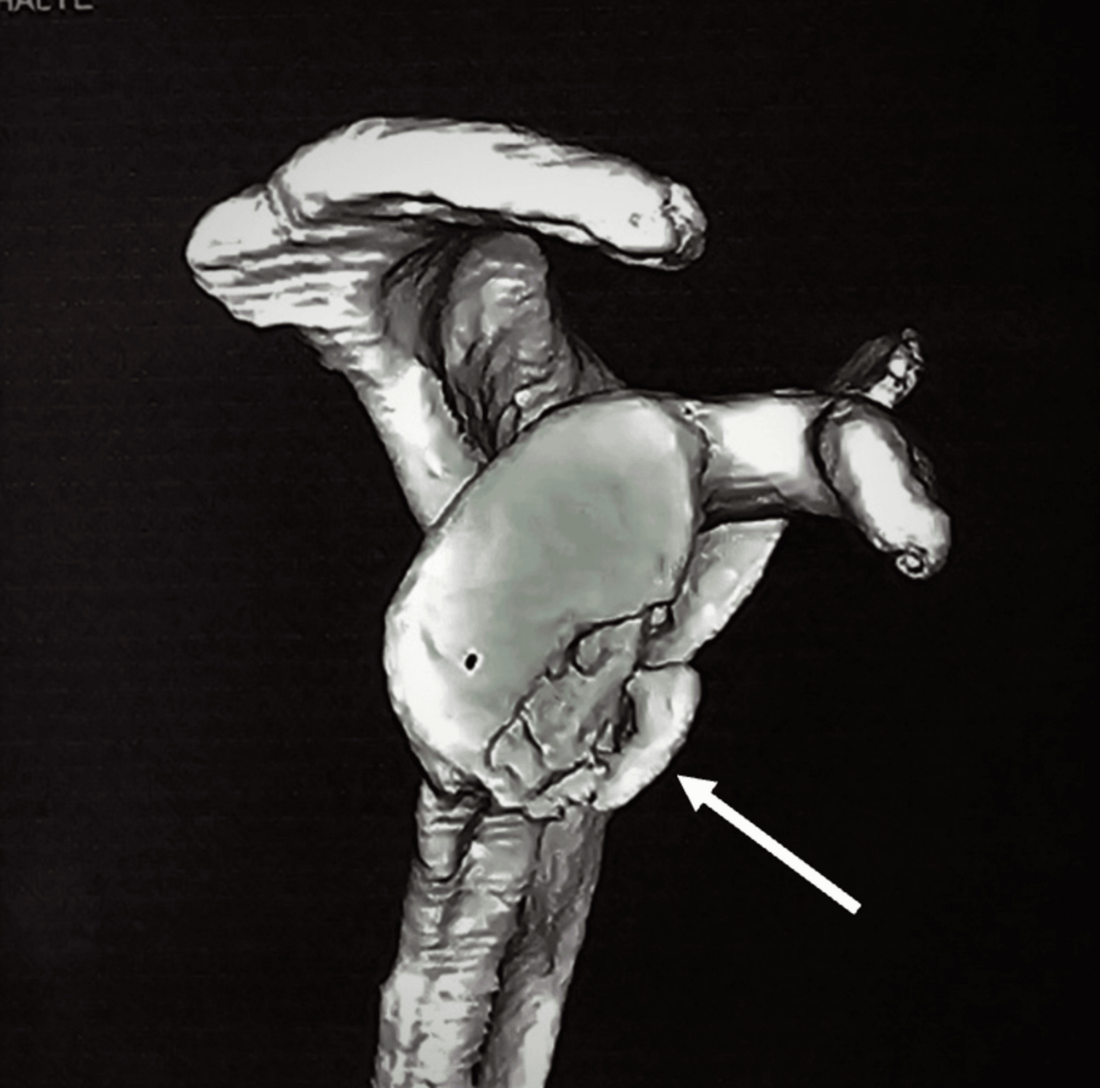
CT images showing the enface view of the scapula with an anterior inferior rim fracture of the glenoid with less than 15% bone loss with depressed osseous fragment (inidcated by the white arrow).
